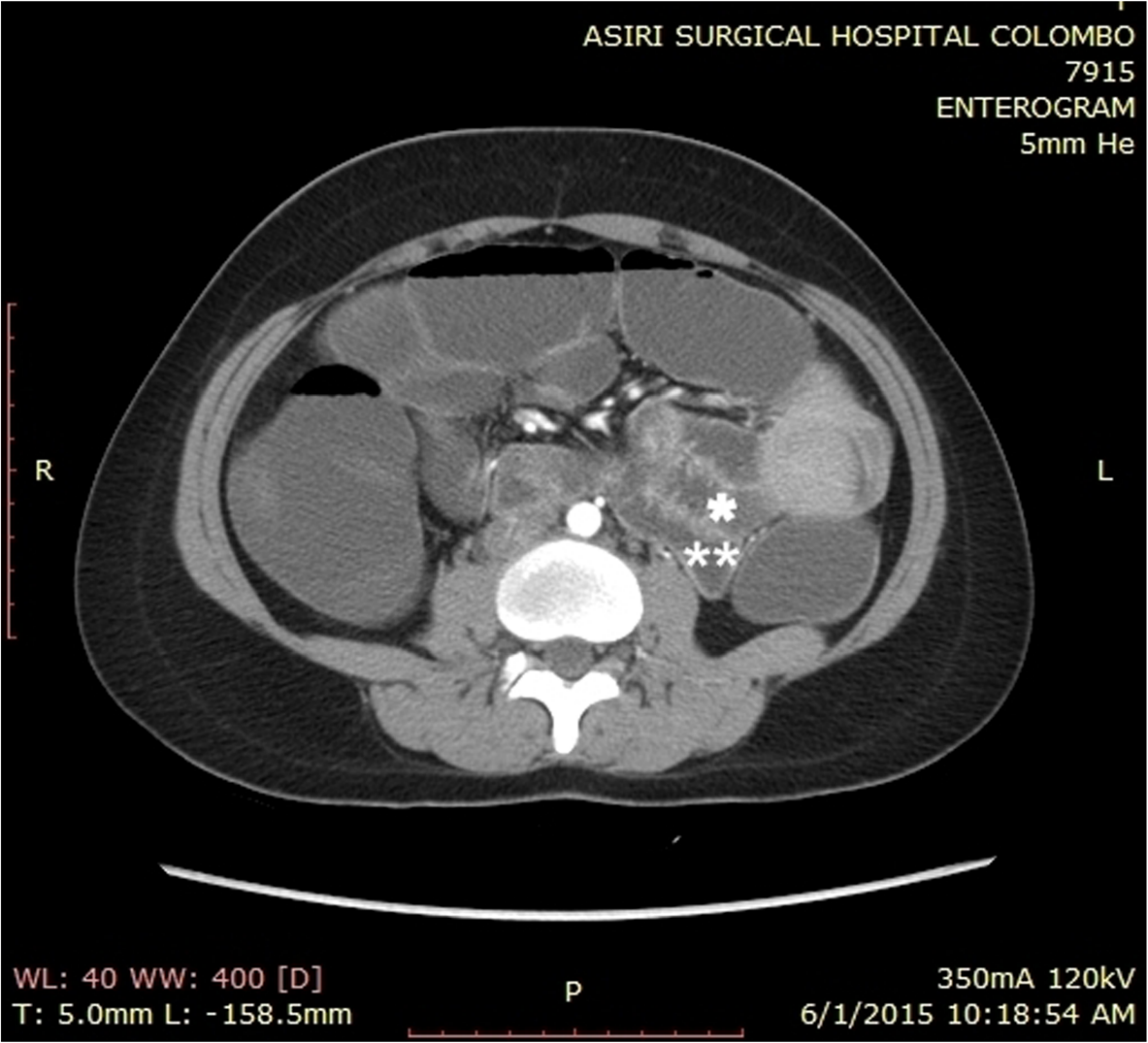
An axial section of the computed tomography enterogram showing the duodenal mucosal intussusceptum (*) and the intussusceptiens – the proximal jejunum ()
